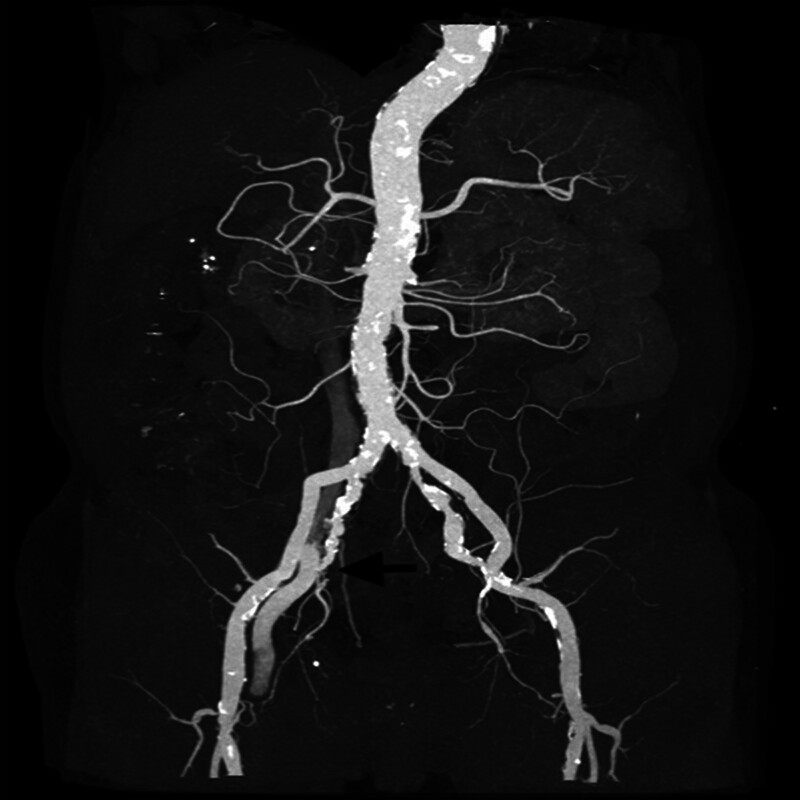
CTA revealing the arteriovenous communication between the right internal iliac artery and the right common iliac vein. CTA = computed tomography angiography.
